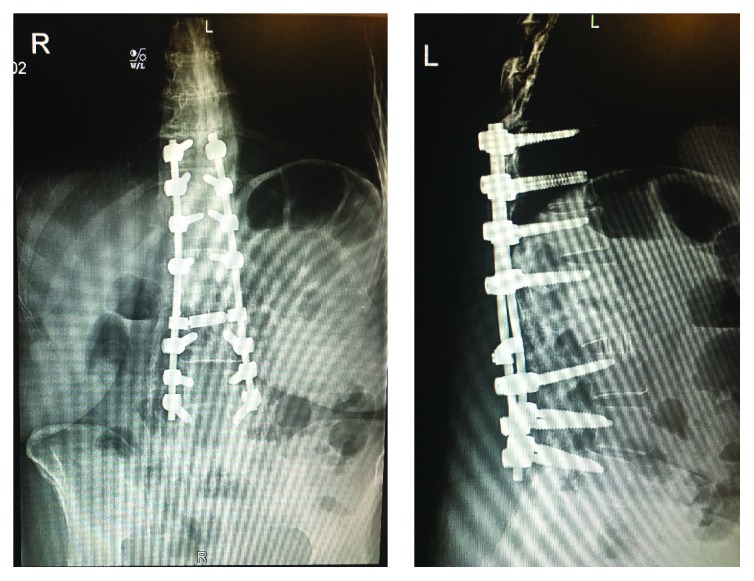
AP and lateral X-ray views at 2-week postoperative visit show T10-L5 posterior segmental instrumentation with evidence of interbody fusion with cage at L2-L3. Evidence of prior fusion at L2-S1 noted. New laminectomy at L1-L2 with prior lumbar decompression noted distally.
